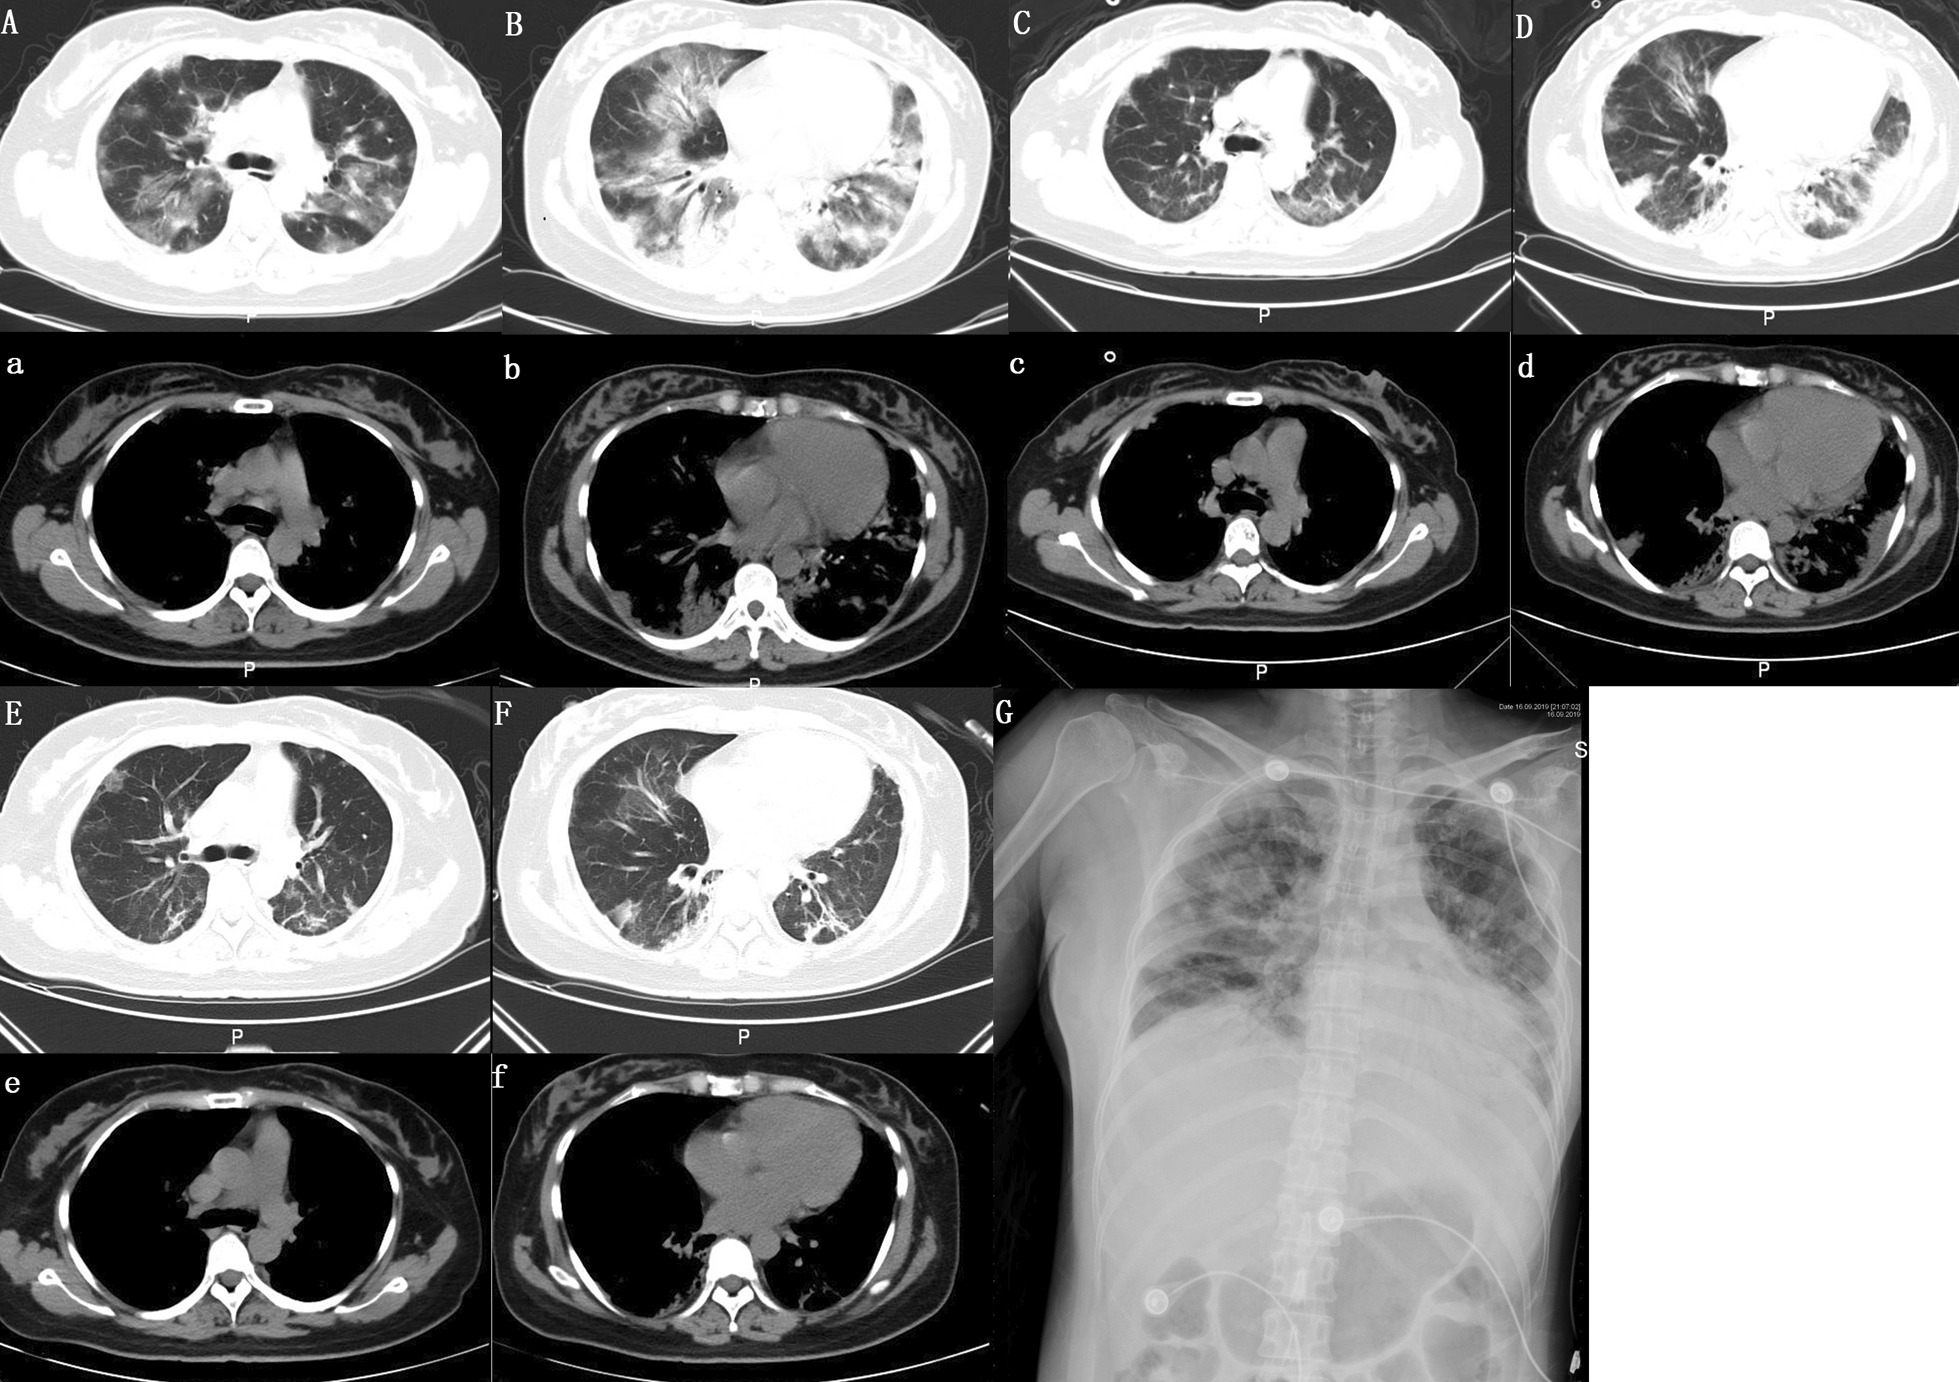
Computed tomography (CT)/X-ray images of the chest of patient 1. Uppercase letters indicate lung windows; lowercase letters indicate mediastinum. A, B, a, b Lung CT on the day of admission; G bedside chest X-ray at 5 days; C, D, c, d lung CT at 13 days; E, F, e, f lung CT at 17 days
